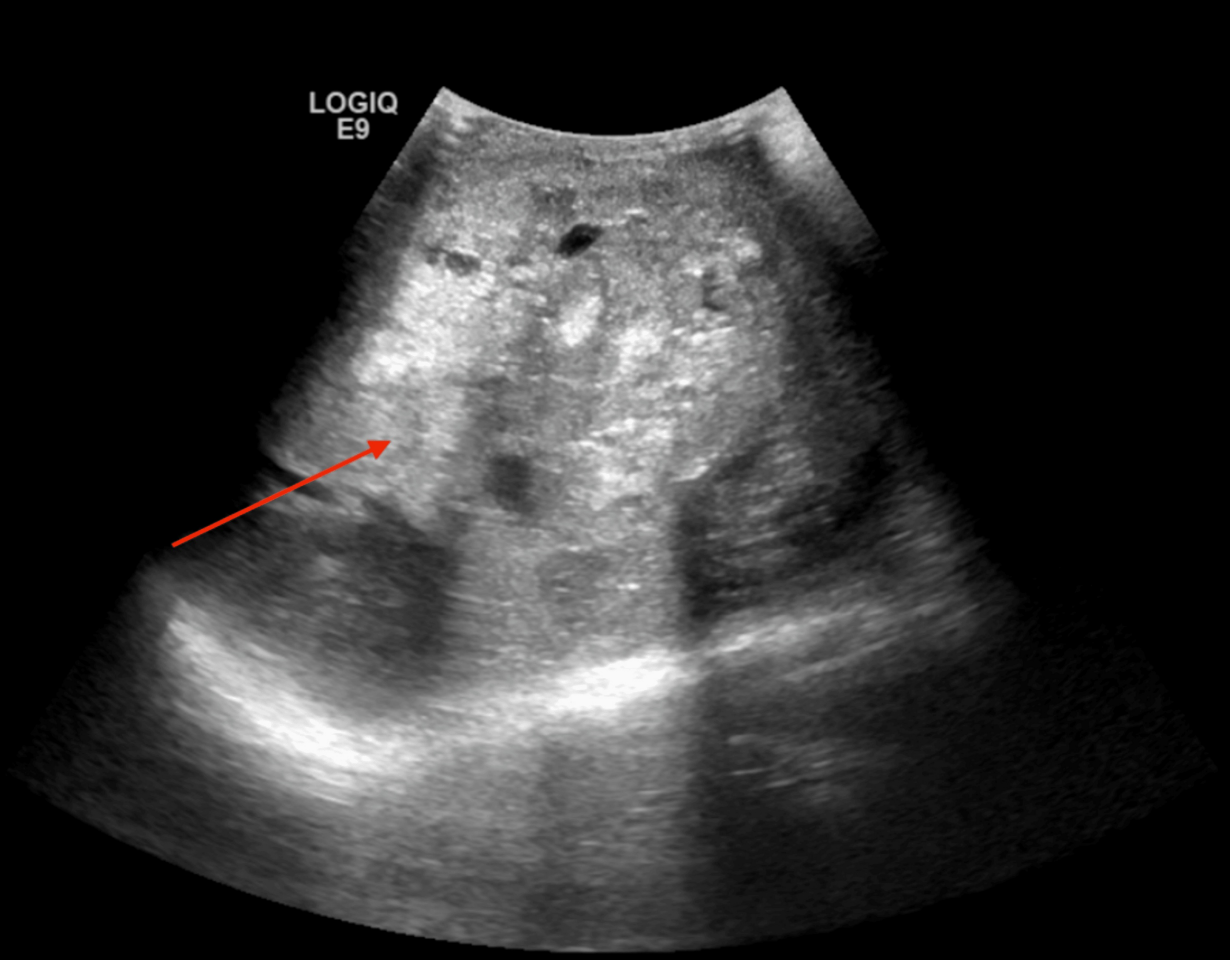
Ultrasonography (US) of the scrotum showing a large mass in the left testicle distending the left scrotum and containing internal vascularity, calcifications, and necrosis, suspicious of a malignant mass (red arrow).
